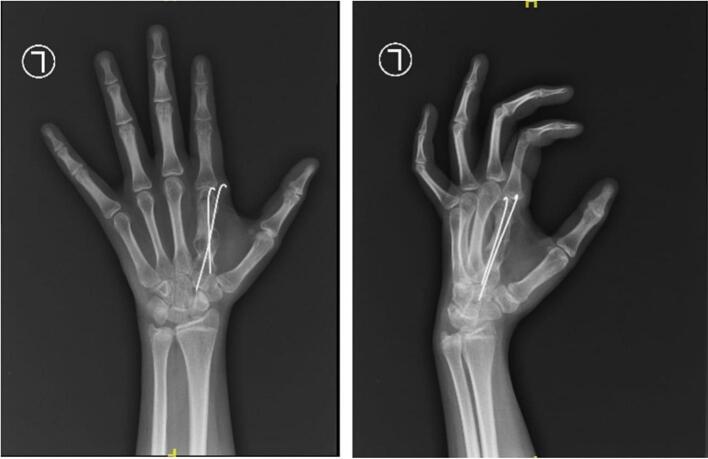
3-month follow-up, we conducted evaluation with X-ray, and there were no signs of recurrent GCT.
